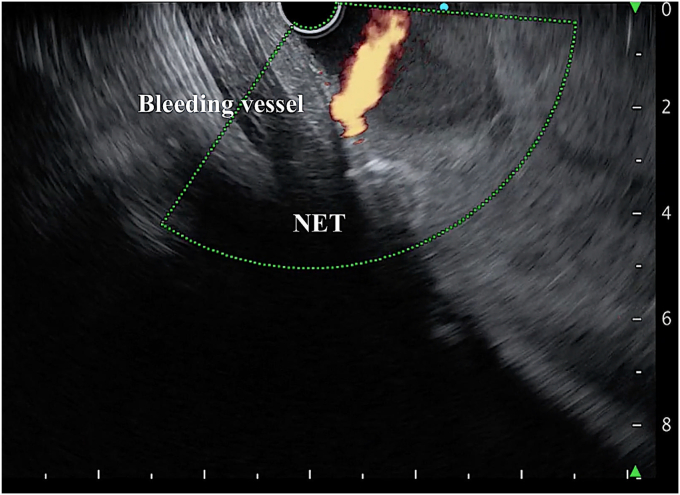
EUS image showing a well-defined NET and adjacent strong Doppler flow from the gastric wall into the lumen suggestive of active bleeding from the shivered submucosal vessel. *NET*, Neuroendocrine tumor.
